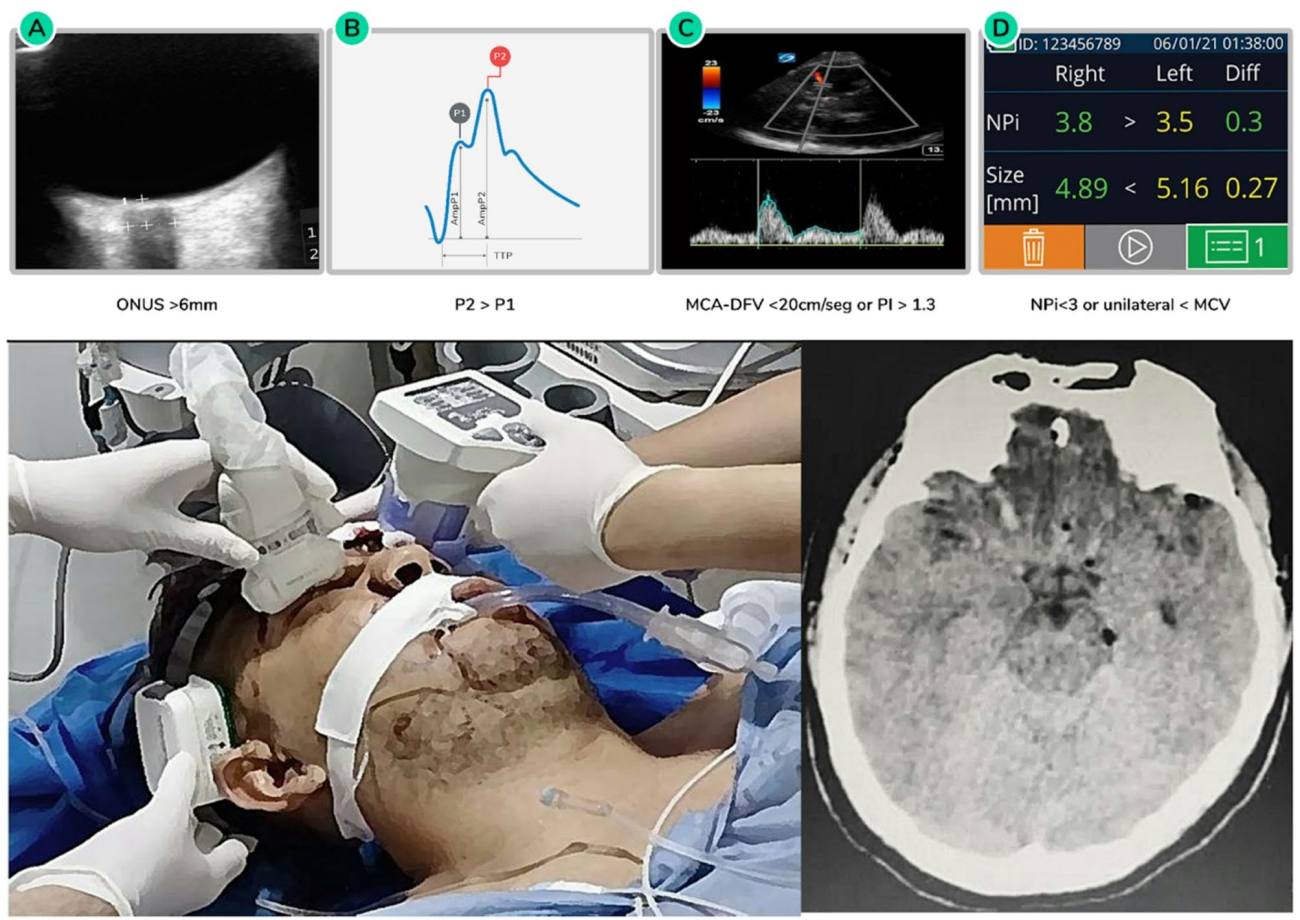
Noninvasive evaluation of intracranial compliance (ICC) in the emergency setting within the MK 4.0 paradigm shift. A severe TBI patient presenting a Marshal II injury at admission. ONSD ultrasound, noninvasive ICP waveform analysis and TCCD (A, B and C respectively) performed in the ER were indicative of ICC impairment, whereas NPi (D) was still favorable. Noninvasive assessment of an impending neurological deterioration. ER: emergency room, ICP: intracranial pressure, ONSD optic nerve sheath diameter, TCCD: transcranial color coded duplex, TBI: traumatic brain injury
